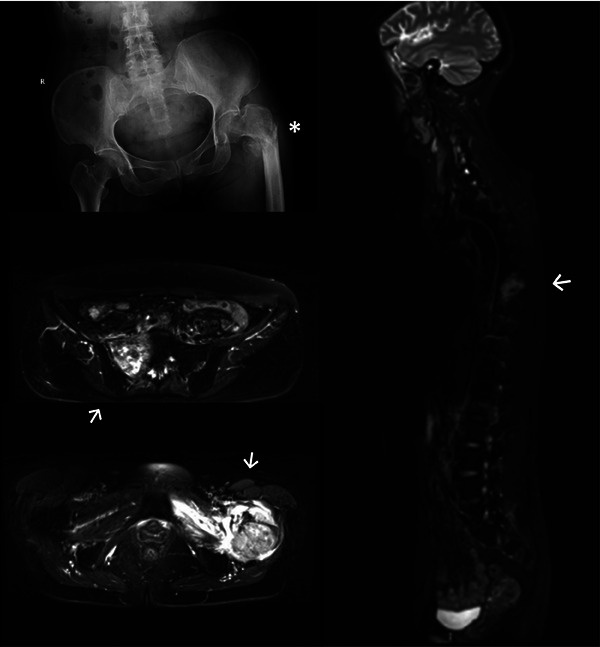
Digital radiography and magnetic resonance images of the bone fracture (*) and metastasis (→).
